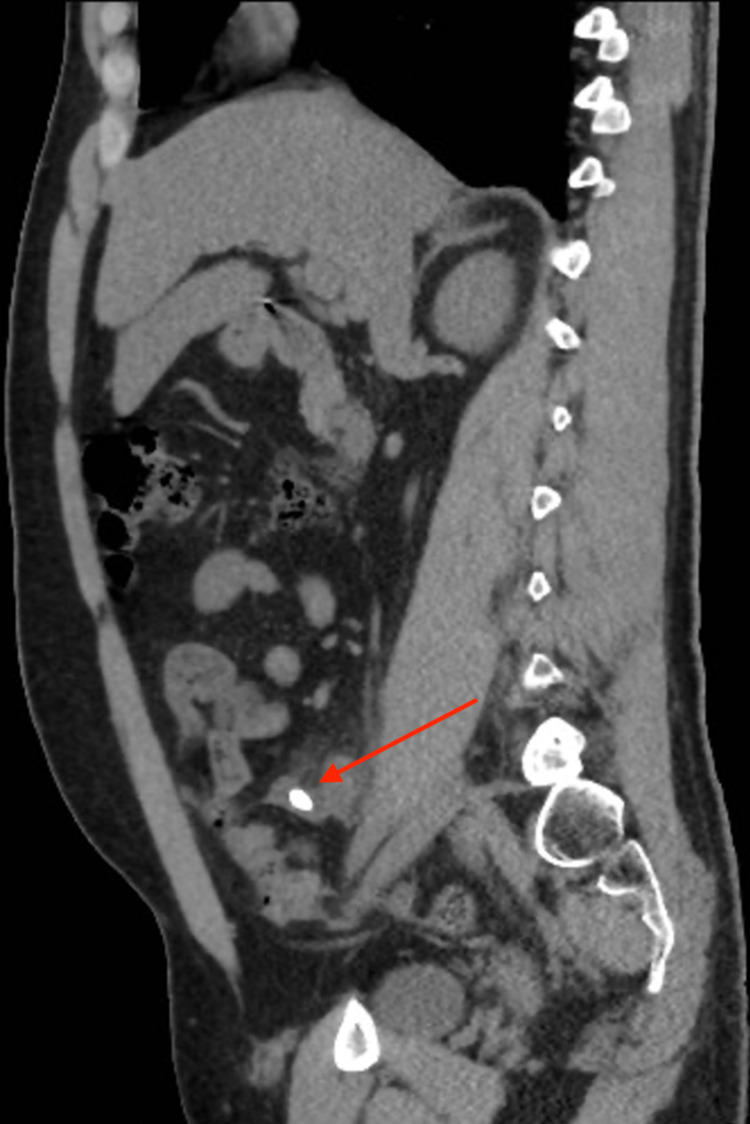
Sagittal CT image of the abdomen and pelvis without contrast Sagittal cut of the CT scan shows an appendicolith within the appendiceal residual tissue with subsequent dilatation of the appendix.
